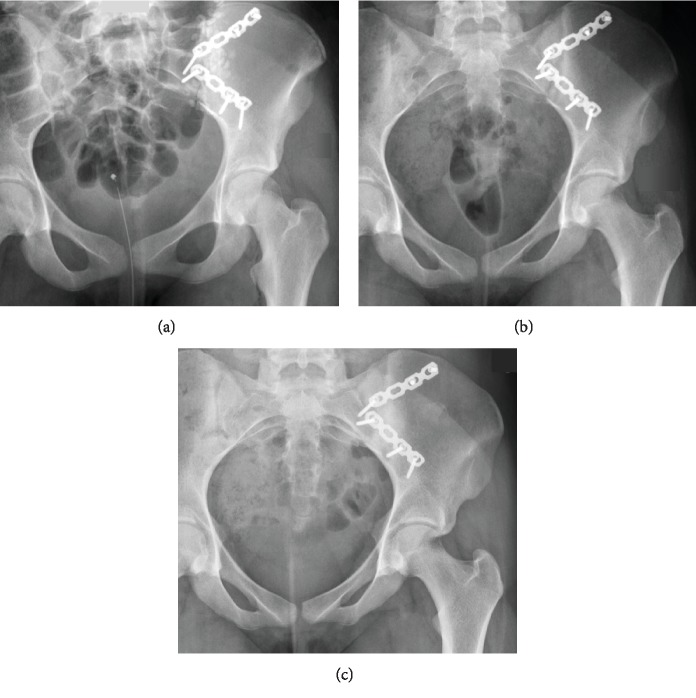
Postoperative anteroposterior pelvic radiograph showing the SI-joint arthrodesis using two plates with radiographically visible Vancomycin-loaded calcium sulphate pellets (a). Three months after surgery (b), the calcium sulphate pellets are totally resorbed. There are no signs of secondary displacement of the SI-joint, implant loosening, or failure. One year after surgery (c), the SI-joint is completely fused.
